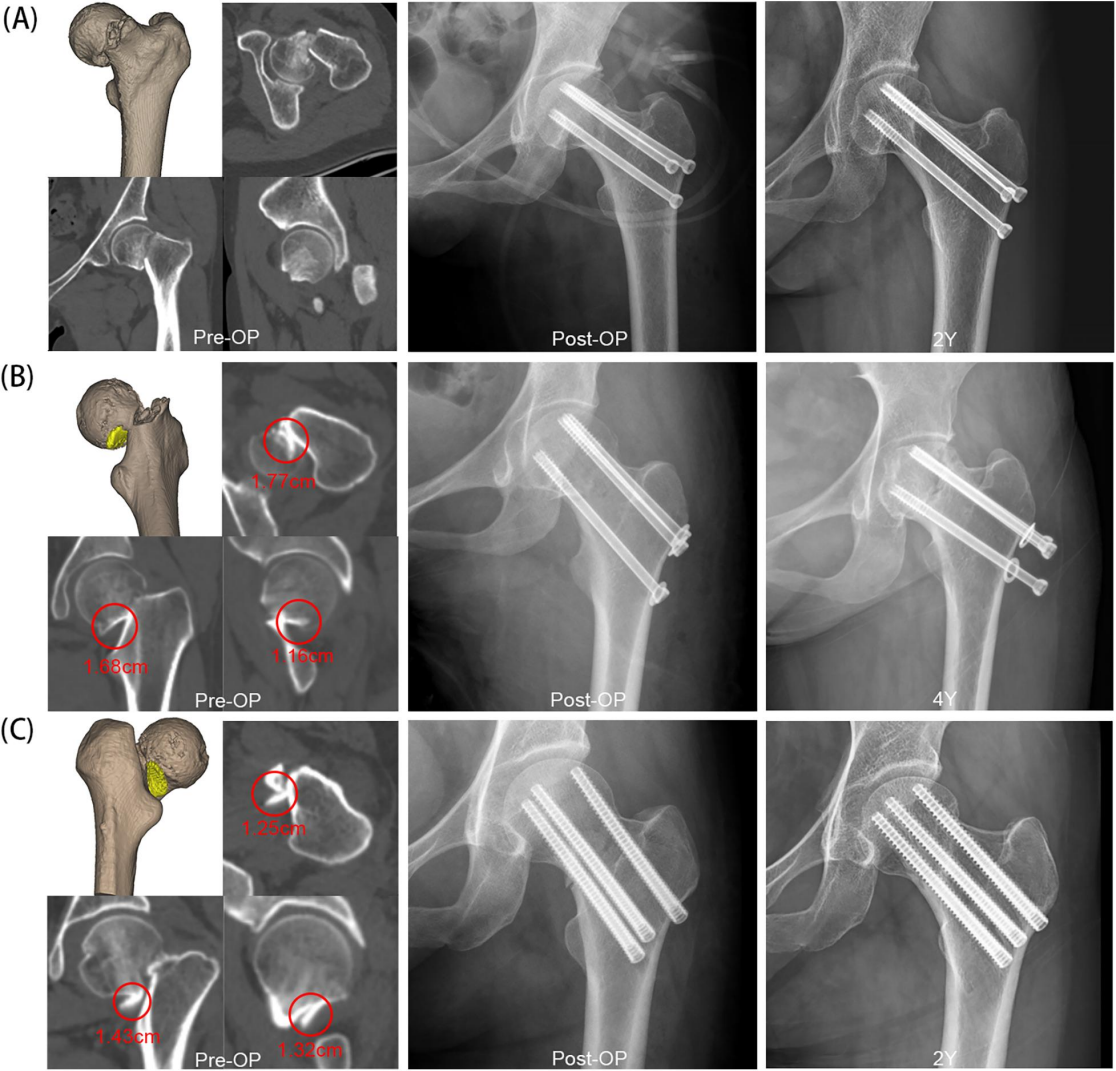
Preoperative and postoperative radiographs of femoral neck fractures treated with three cannulated screws. The fragmented debris are circled, with their maximum diameter indicated. (A) A 50-year-old woman with a non-comminuted femoral neck fracture exhibited good radiographic healing two years after fixation with partially threaded screw fixation; (B) A 34-year-old woman with a comminuted femoral neck fracture developed severe femoral neck shortening and avascular necrosis of the femoral head four years after undergoing partially threaded screw fixation; (C) A 53-year-old woman with a comminuted femoral neck fracture exhibited good radiographic healing two years after fixation with fully threaded screws.
